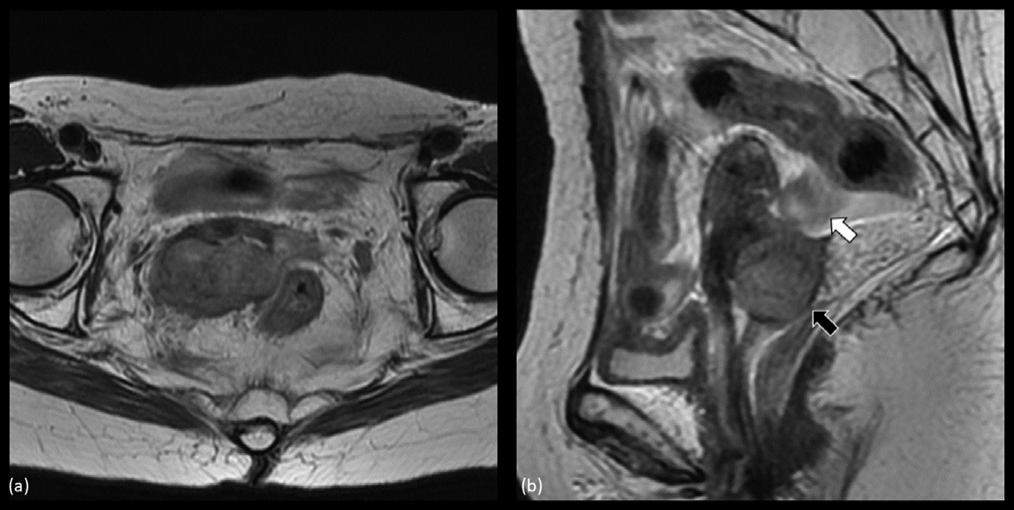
MRI of recurrent cervical cancer before salvage brachytherapy. (a) shows an
